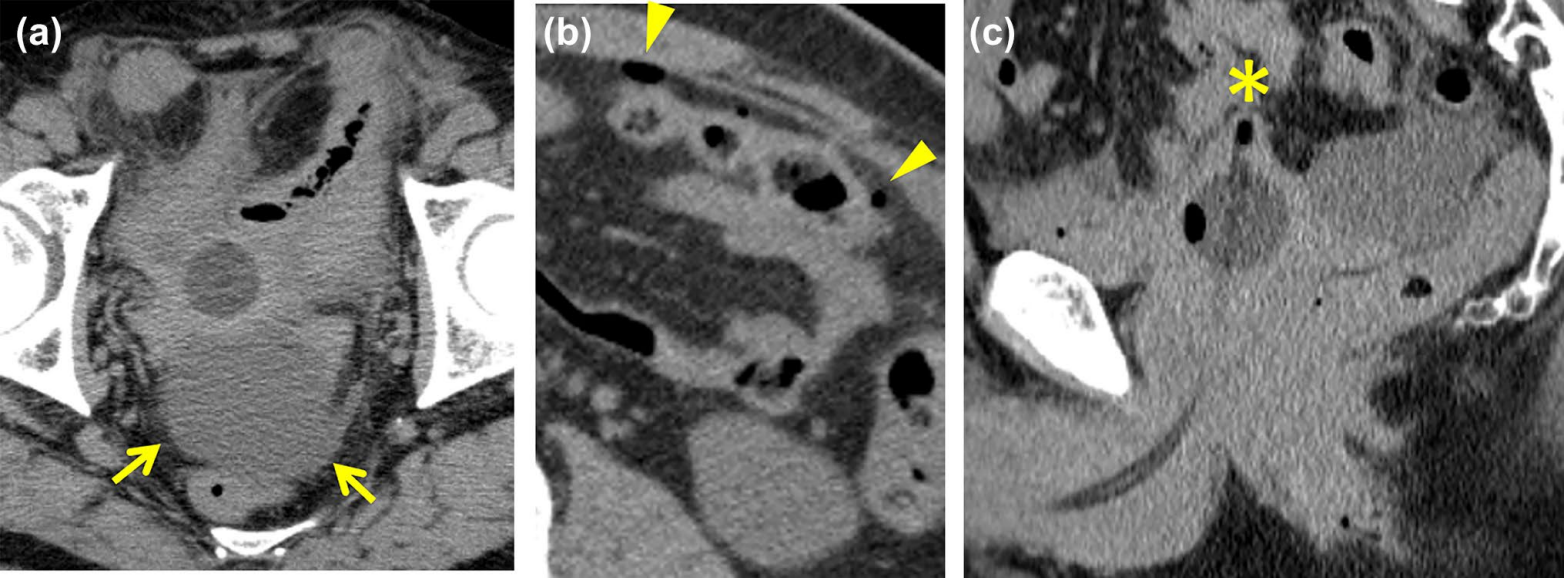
Pitfalls. 70-Year-old man with perforation of the small intestine who presented with urinary retention and abdominal pain. a b Axial NECT images obtained after Foley catheter placement demonstrate ascites (arrows) and extra-luminal gas (arrowheads). The CT value of ascites is relatively high (25-30HU). c A sagittal image reveals thinning of the bladder wall around the catheter tip (*), which led to a misdiagnosis of bladder rupture. Subsequent cystography was obtained and ruled out bladder rupture. Exploratory laparotomy revealed perforation of the small intestine.
